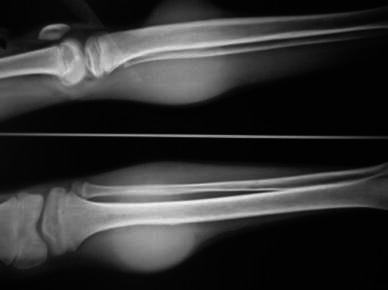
Plain radiograph showing soft tissue involvement with intact cortex
